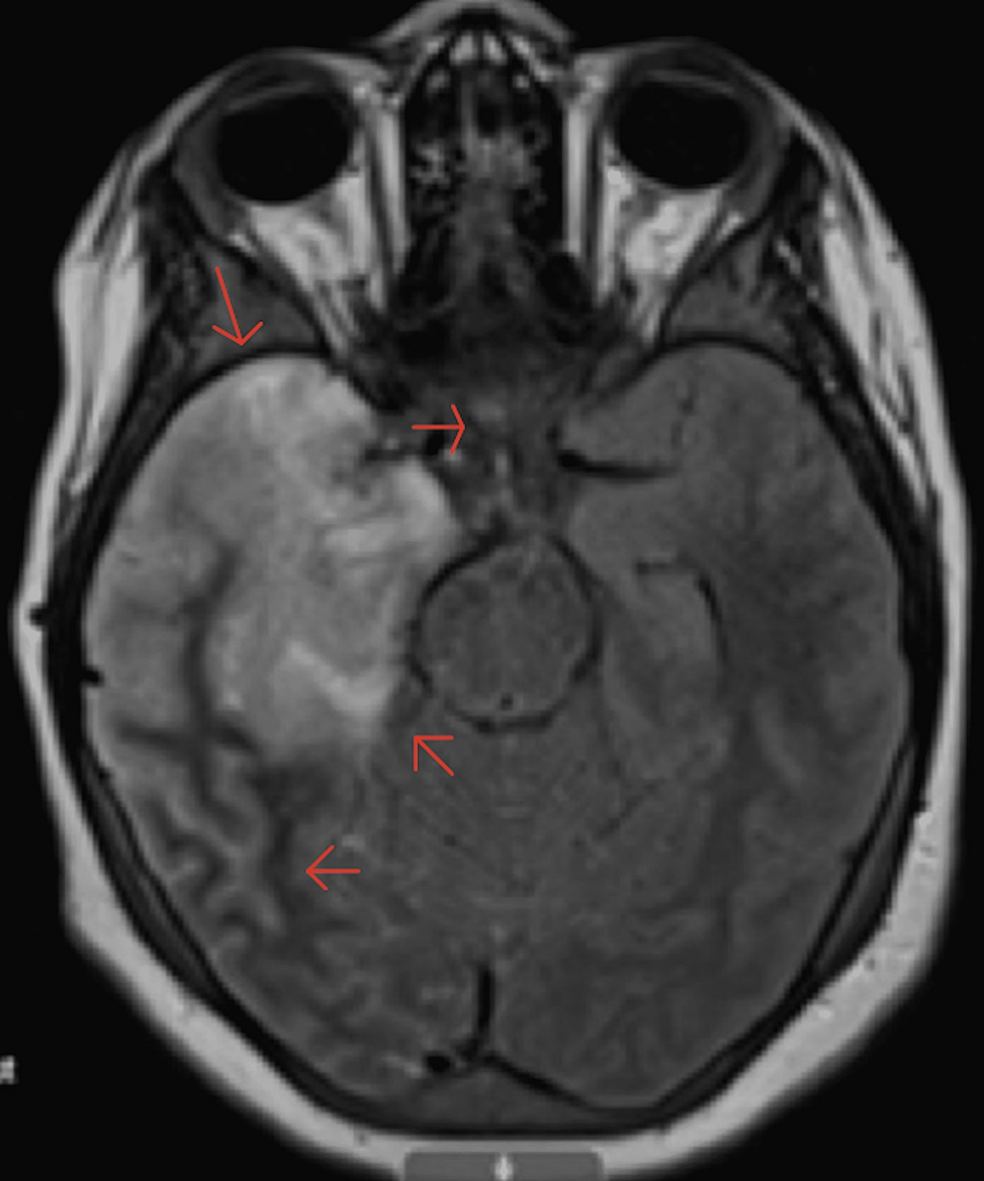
MRI brain without contrast in a T1-weighted axial view MRI brain without contrast in a T1-weighted axial view shows hemorrhagic transformation (red arrows) in the right mesial temporal lobe.
